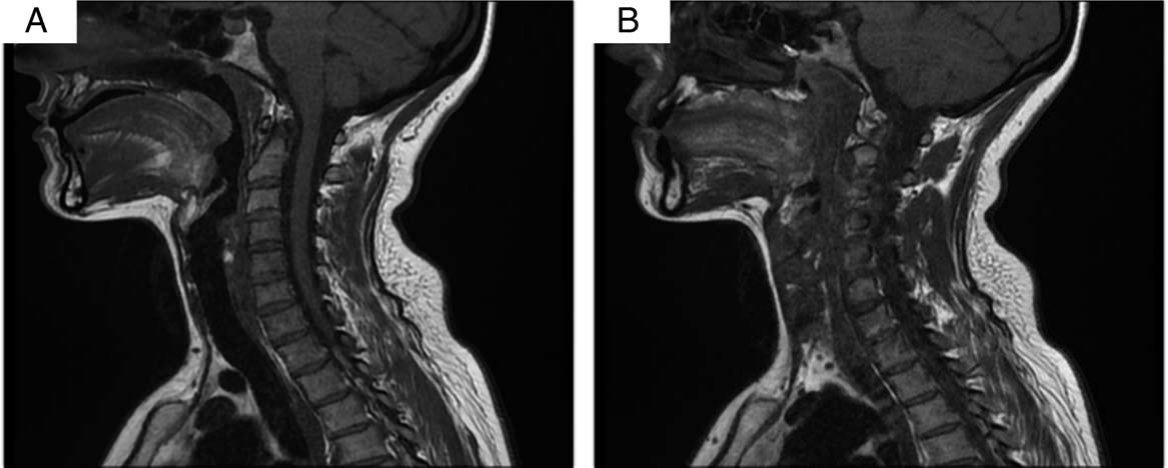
(A) Multiplanar multisequence MRI of the cervical spine with contrast done on 1.5 T showed normal cervical curvature with no significant spinal cord stenosis or neural foramina narrowing. (B) Multiplanar multisequence MRI of the cervical spine with contrast done on 1.5 T showed intact prevertebral and paravertebral soft tissue.
